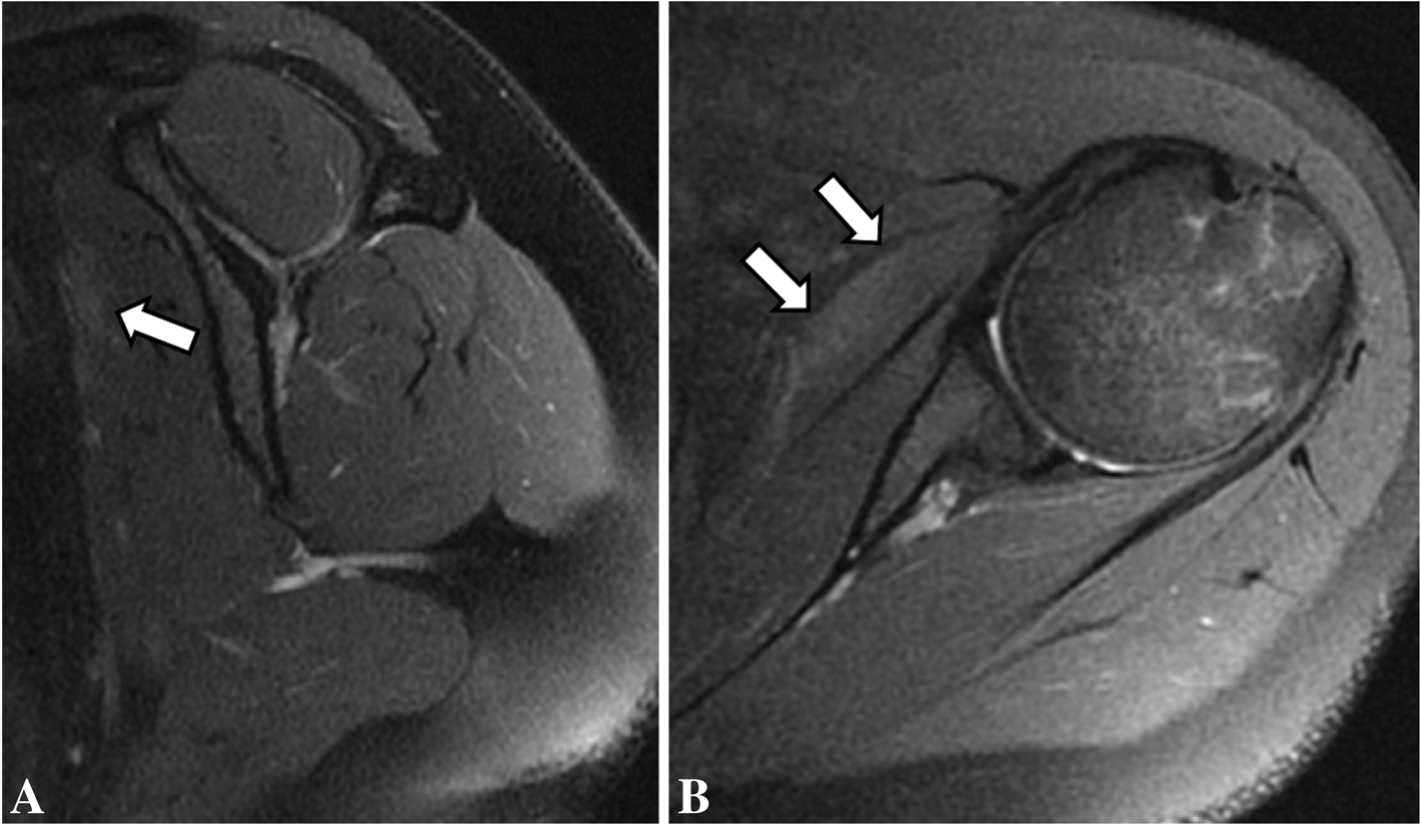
Sagittal (a) and axial (b) T2-weighted fat-suppressed MR images showing mild edema of the subscapularis muscle (arrows)
